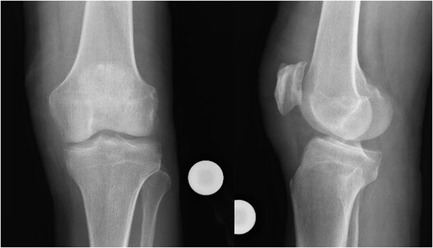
Left knee A‐P and lateral radiographs status postrevision quadriceps tendon repair with biologic augmentation show normalization of patellar heights with a Caton‐Deschamps index of 0.91. (A‐P, anterior‐posterior.)
